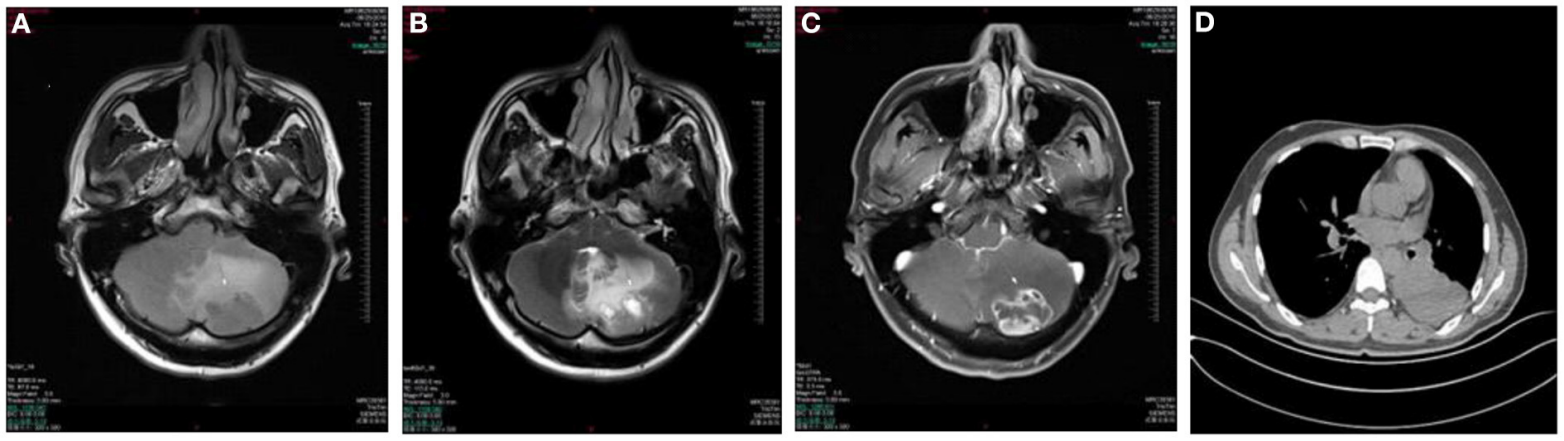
Case 6: metastasis of the left cerebellar hemisphere. Axial T1WI (A) and Axial T2WI (B) show irregular lesion and surrounding edema in the left cerebellar hemisphere with the heterogeneously hypointense signal on T1WI and marked hyperintense signal on T2WI relative to the normal parenchyma of the cerebellum. The lesion shows ring-enhancement on postcontrast axial T1WI with fat saturation (C). Chest CT shows a soft tissue attenuation mass in the lower left lung (D).
